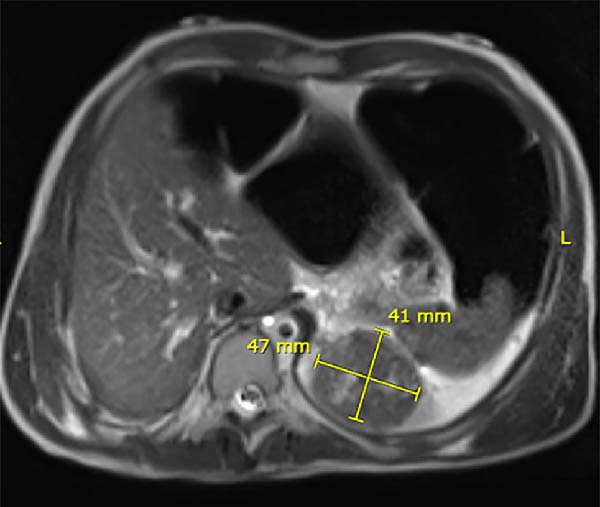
Magnetic resonance image in axial section showing the same splenic mass measuring over 4.5 cm.
